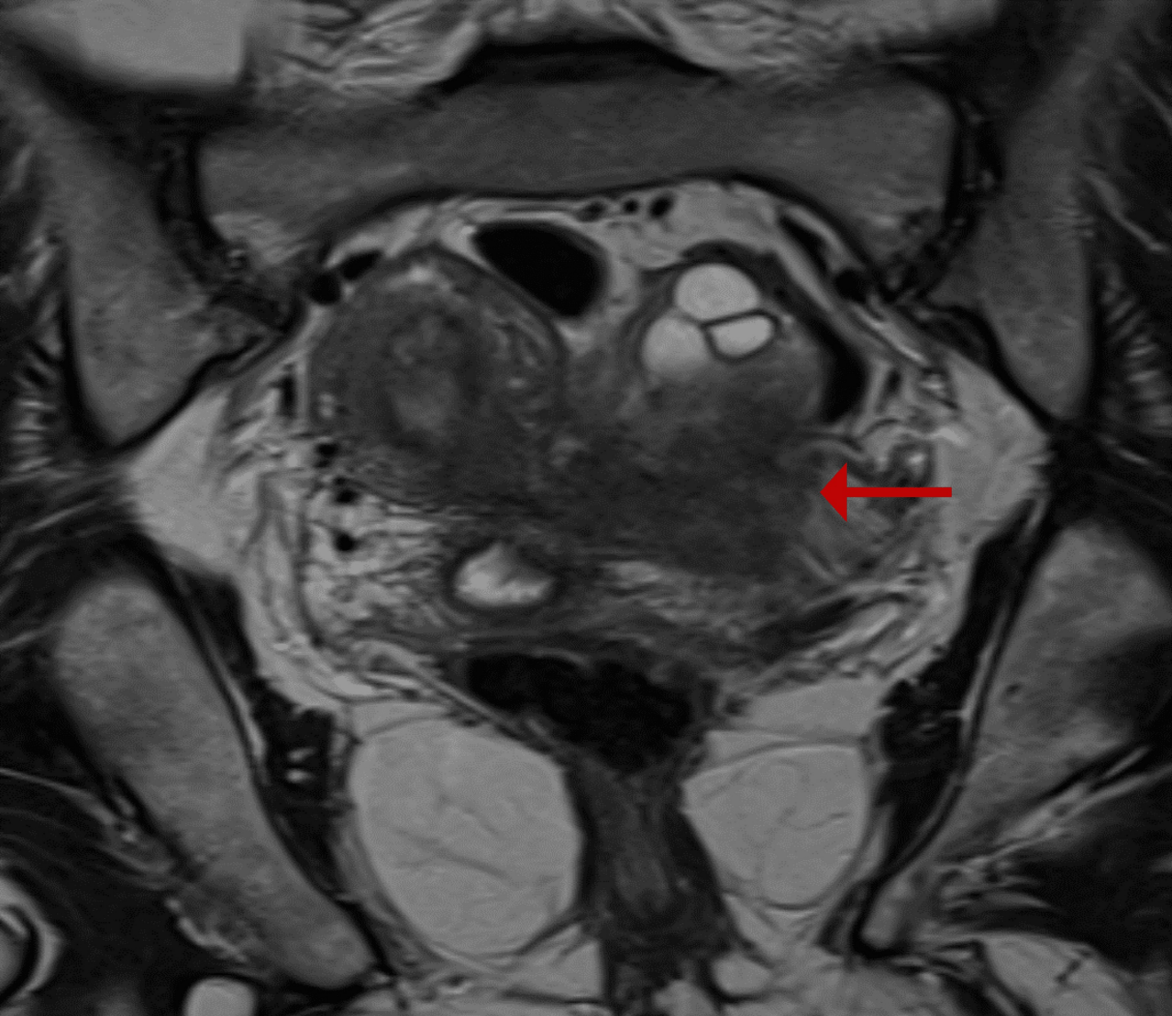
T2 weighted coronal section of MRI pelvis showing rudimentary horn on the left side of the uterus (red arrow).
